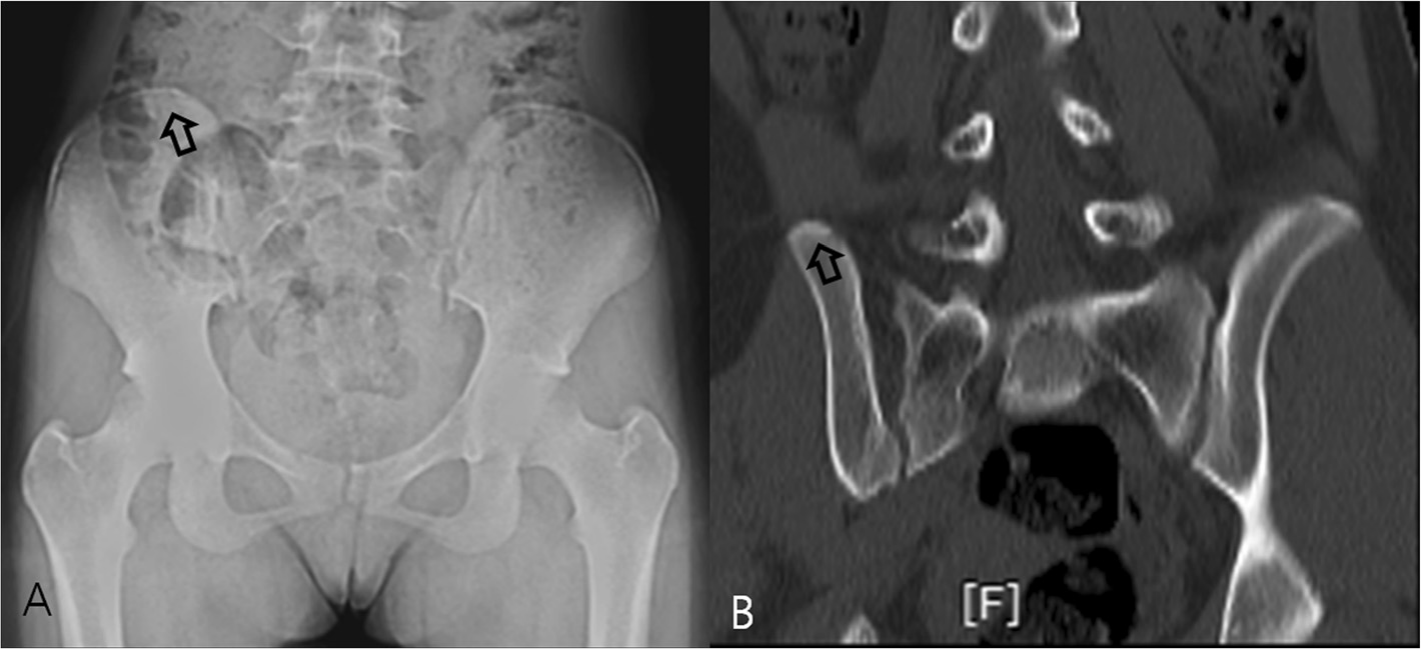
Micro-fusion. (A) Plain radiography of a 14-year-old girl; a radiolucent line was visible between the apophysis and the posteromedial iliac bone and was graded as Original Risser stage 4 and French Risser stage 3. (B) A coronal 3D reconstruction view; there was absence of any radiolucent line indicating definite fusion, and the staging by Original method was 5 and by French method was 4. Accurate estimation of fusion of iliac apophysis or micro-fusion is difficult on plain radiography.
